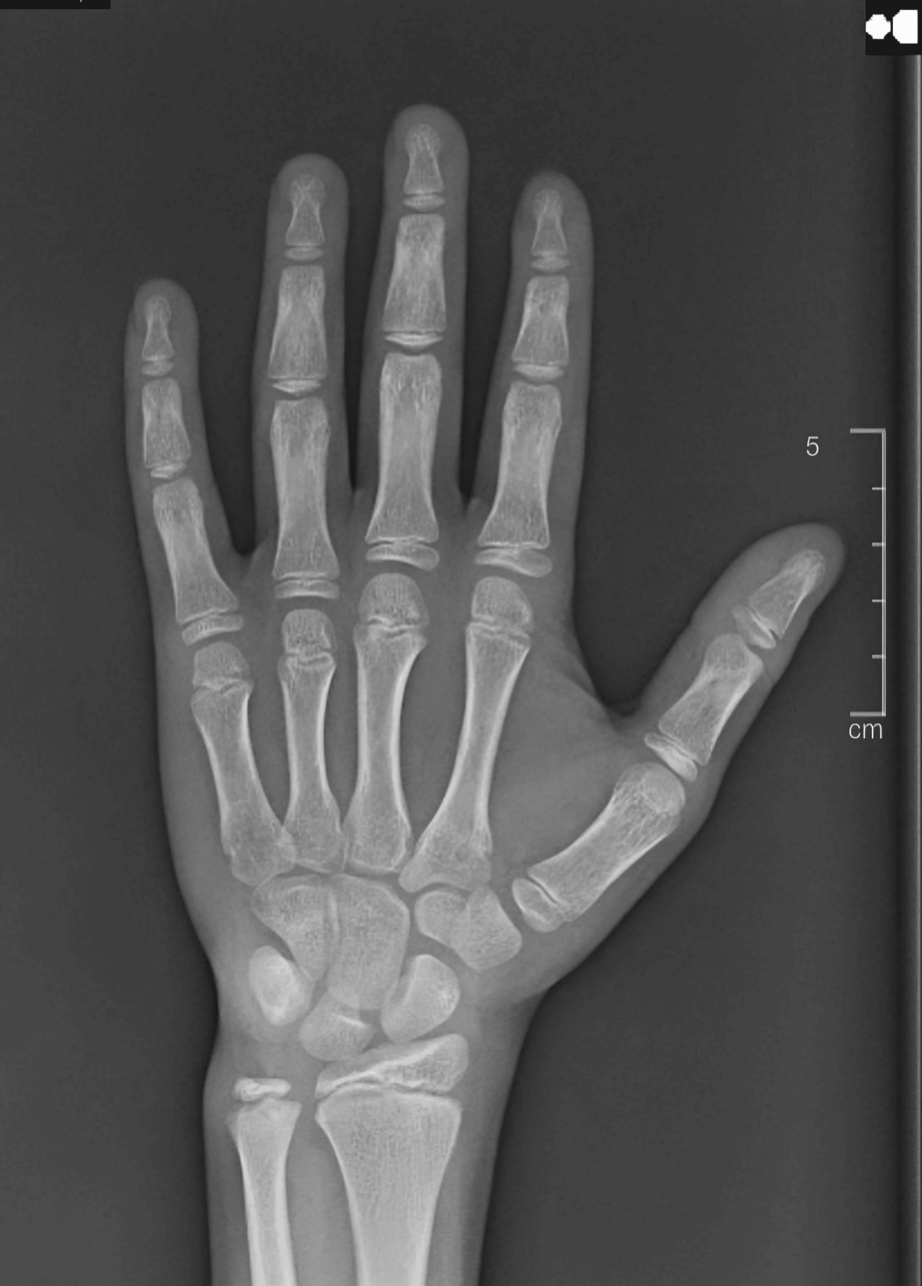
X-ray of the left hand showed a bone age corresponding to 10 years.
